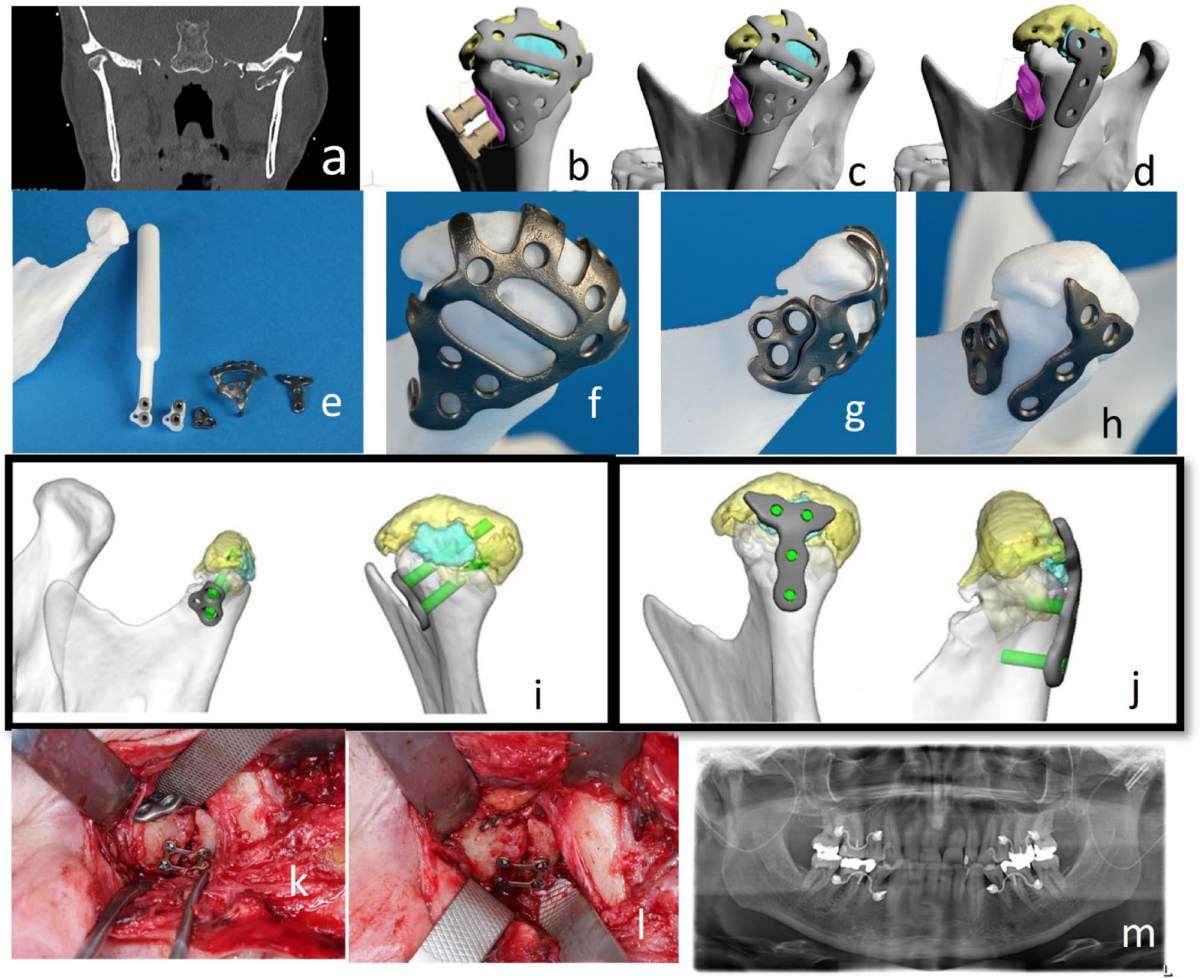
Case 4: Preoperative CT-Scan (representative coronal view) – left mandibular AO type-p CHF with multi-fragmentation (three pieces) and lost vertical apposition (a). VSP/CAD – fracture reduction and PSD components (dorsal view) - large medial dome fragment (yellow), SDIMF (cyan). Dome fragment in unison with SDIMF encompassed by upper portion of primary PSD component; bipartite central window in PSD midportion; lower PSD portion surrounds postero-lateral condylar neck and base transition zone; navigation/washer PSD in its docking position; cylindrical drill guides attached (b). VSP/CAD – primary PSD component plus navigation/washer PSD in key-lock position (c). VSP/CAD – finally remaining PSD components: primary PSD removed, navigation/washer PSD in situ, T- shaped PSD over posterior condylar surface retaining SDIMF (d). Overview – STL-model (left condylar process reduced), 2 drill guides, 3 distinct PSDs (from left to right) for reduction/prefixation, navigation/washer function and fragment retention/ reinforcement (e). STL-model – dorsal view of reduced CHF with reduction/prefixation PSD component (f). Same STL-model – lateral view after tongue-in-groove docking of navigation/washer PSD and reduction/prefixation PSD (g). Same STL-model – dorso-lateral view with primary PSD removed, navigation/washer PSD left in place, additional T-shaped retaining/ reinforcement PSD (h). VSP – navigation/washer PSD placed under LSFPSO; intramedullary LSFPSO pathways (green) (i). VSP – T-shaped PSD fixated with small diameter screws (green) (j). Intraoperatively – temporary fixation of medial condylar head fragment with conventional grid plate; navigation/washer PSD fastened (k). Same view after removal of the washer and insertion of 2 lateral screws (l). Postoperative Panorex (m)
